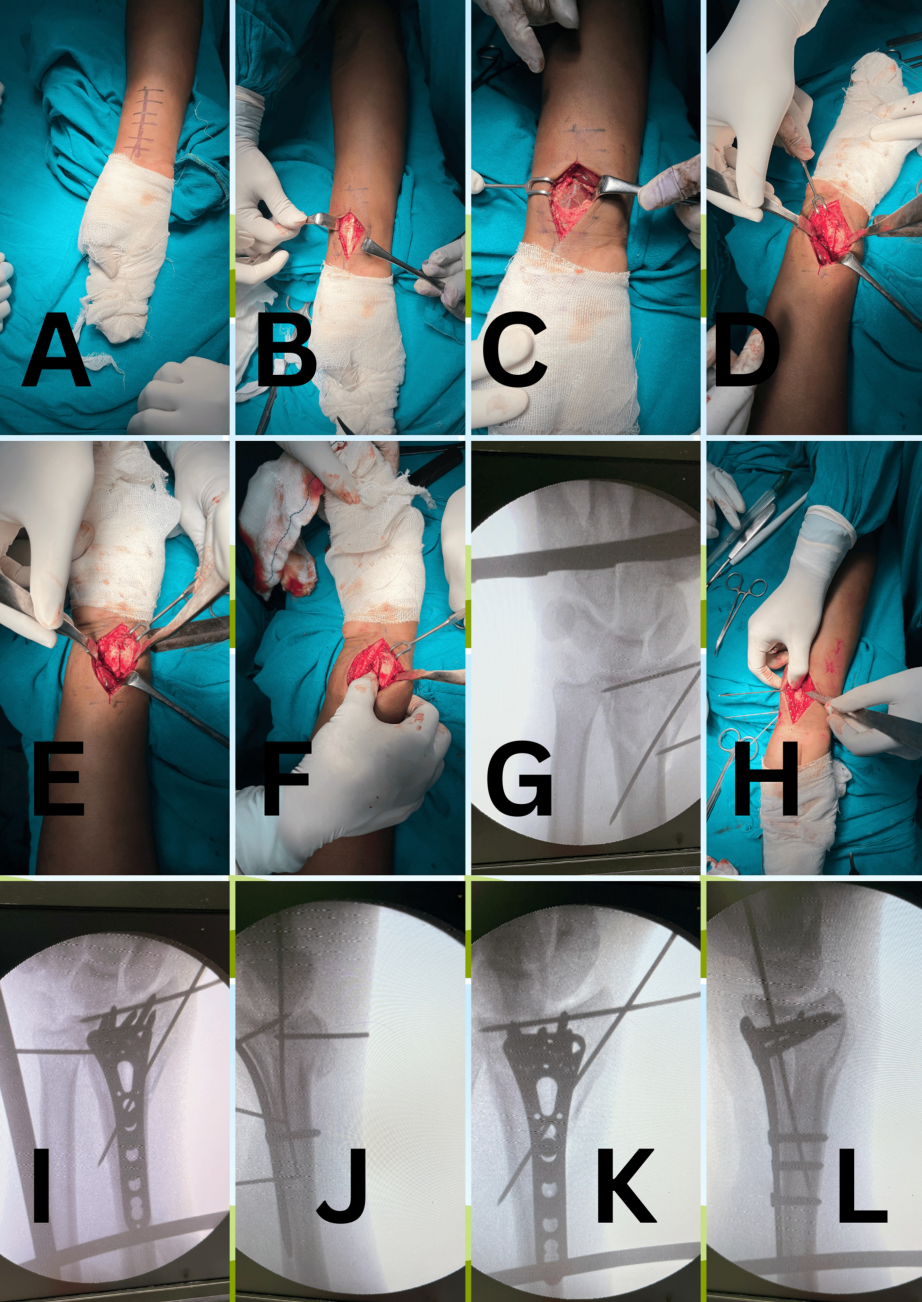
Technique of three-column distal radius fracture fixation with volar locking plate and K-wires through a single incision (A) Patient positioning and incision marking. (B) FCR tendon visible through the incision. (C) Pronator quadratus muscle exposed after retracting the FCR tendon. (D) Radial sigmoid notch and fracture fragments visible through the incision. (E) Middle column fracture visible. (F) Radial column and radial styloid fully exposed. (G) Fracture fragments temporarily fixed with multiple 2.0-2.5-mm K-wires (AP view X-ray). (H) Fracture fragments temporarily fixed with multiple 2.0-2.5-mm K-wires. (I) AP X-ray after volar plate fixation with K-wires. (J) Lateral X-ray after volar plate fixation with K-wires. (K) Final AP X-ray showing volar plate fixation with K-wire in radial styloid and sigmoid notch.
